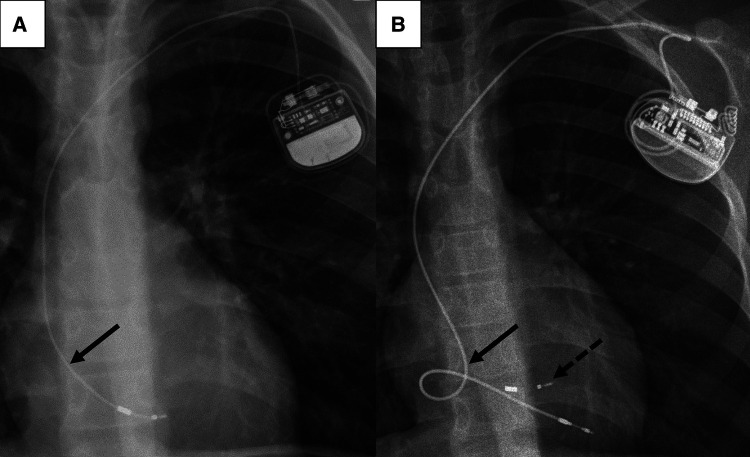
Chest radiographs of a 12-year-old boy before (A) and after (B) TLE. (A): depleted length reserve (arrow) of the right ventricular lead due to somatic growth. (B): lead length reserve for anticipated further somatic growth of the new lead implanted after TLE. The lead “reserve loop” is positioned in the right atrium (arrow). A fragment of the lead tip from the previously implanted 4472 model (Boston Scientific Fineline™ II) lead (dotted arrow) remained in the right ventricle. TLE, transvenous lead extraction.
